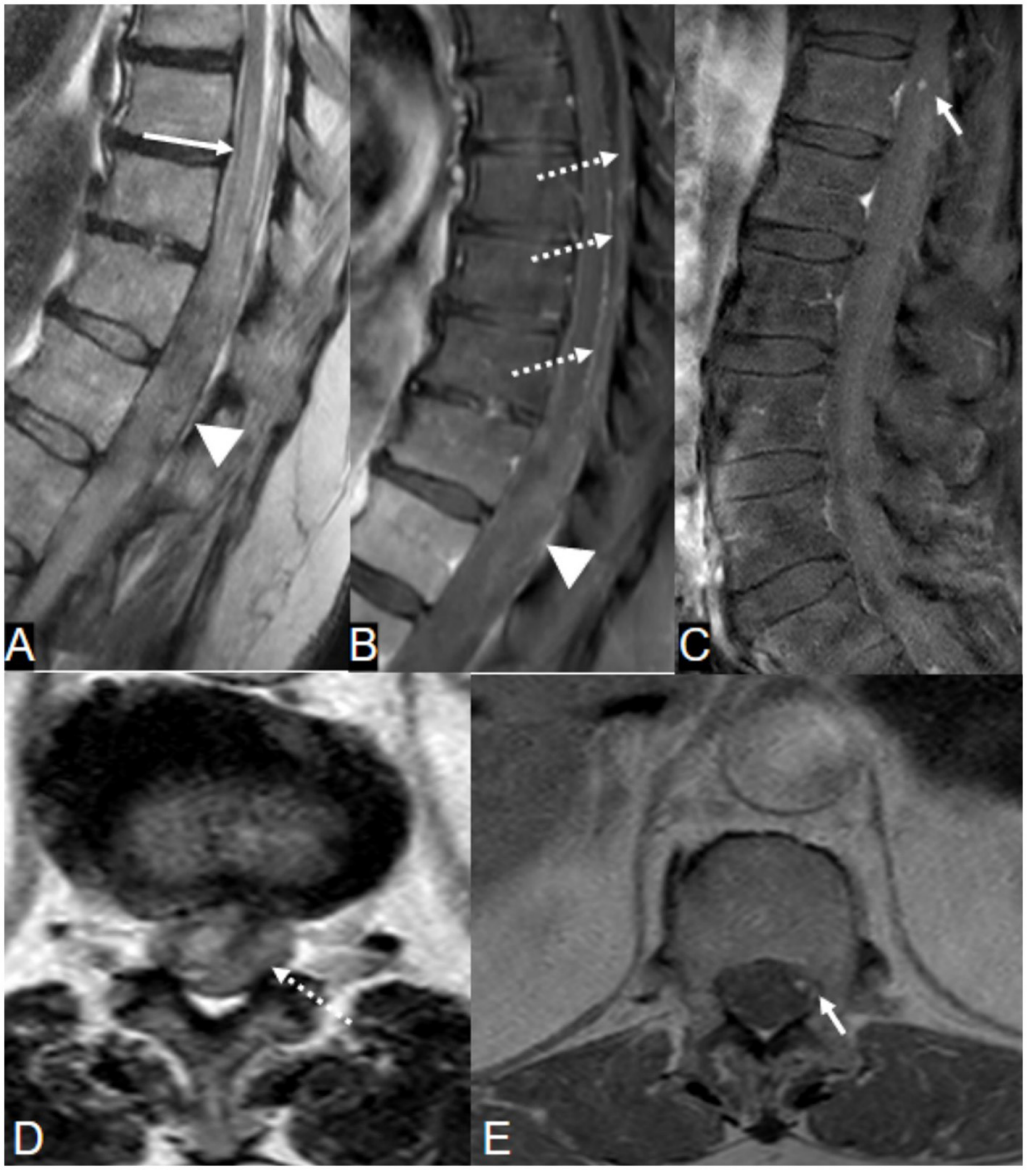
A 66-year-old woman on warfarin presented with acute severe low back pain. (A, B, D) T2-weighted MRI images demonstrate conus edema (long arrow) with spinal subdural hematoma, predominantly on the left, displacing the conus to the right (arrowheads). Note the lateral compression of the spinal cord and cerebrospinal fluid by the hematoma (long arrowhead). (C, E) Contrast-enhanced MRI shows focal nodular enhancement at the left peripheral aspect of the cord at the T12 level (short arrow), confirmed later on angiogram as a ruptured left T12 posterior spinal artery dissecting aneurysm (Figure 3A).
